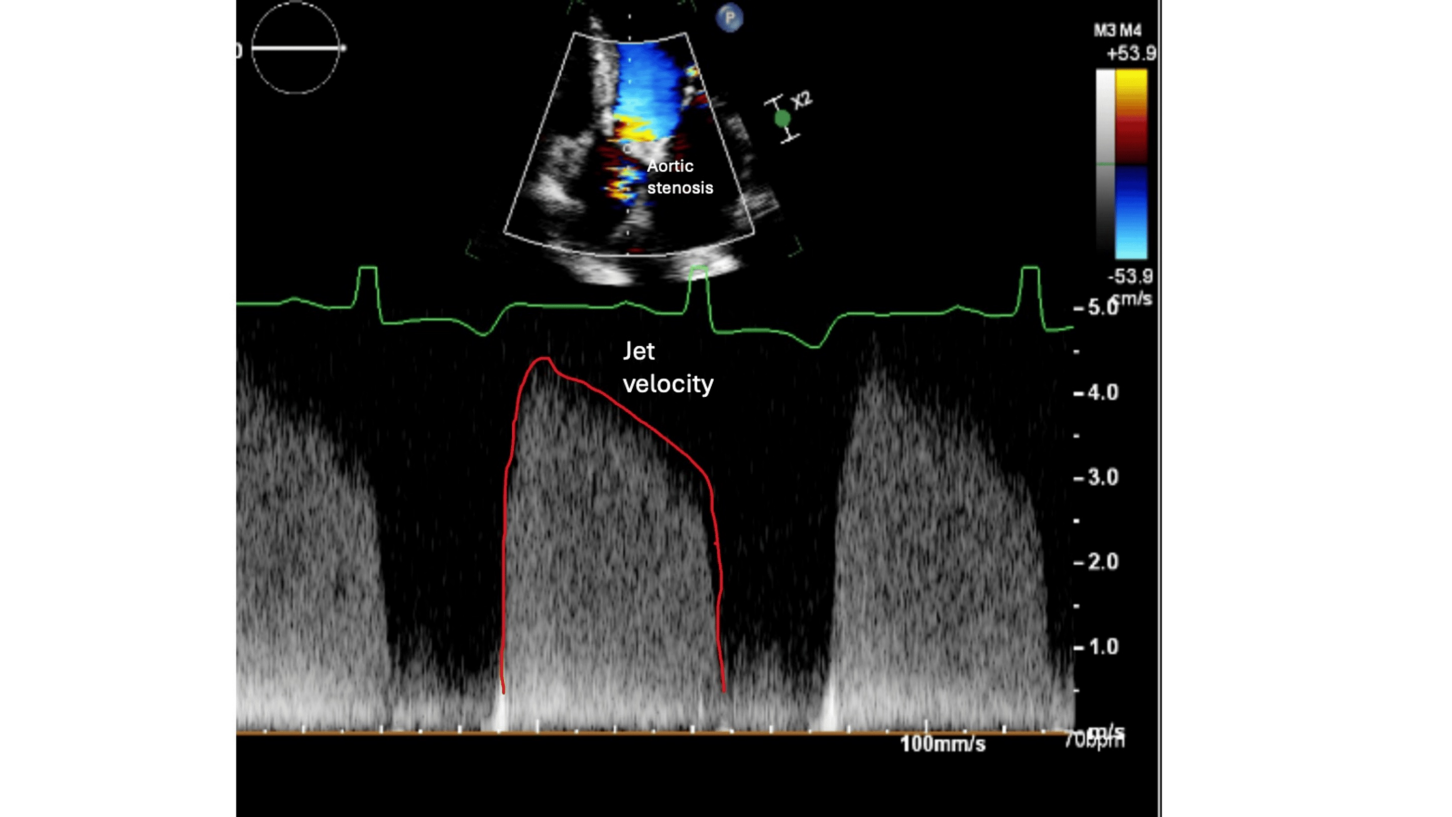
Transthoracic echocardiography image showing jet velocity across the aortic valve The increased velocity suggests significant aortic stenosis, which, in conjunction with the regurgitation observed, points to severe mixed valvular disease.
